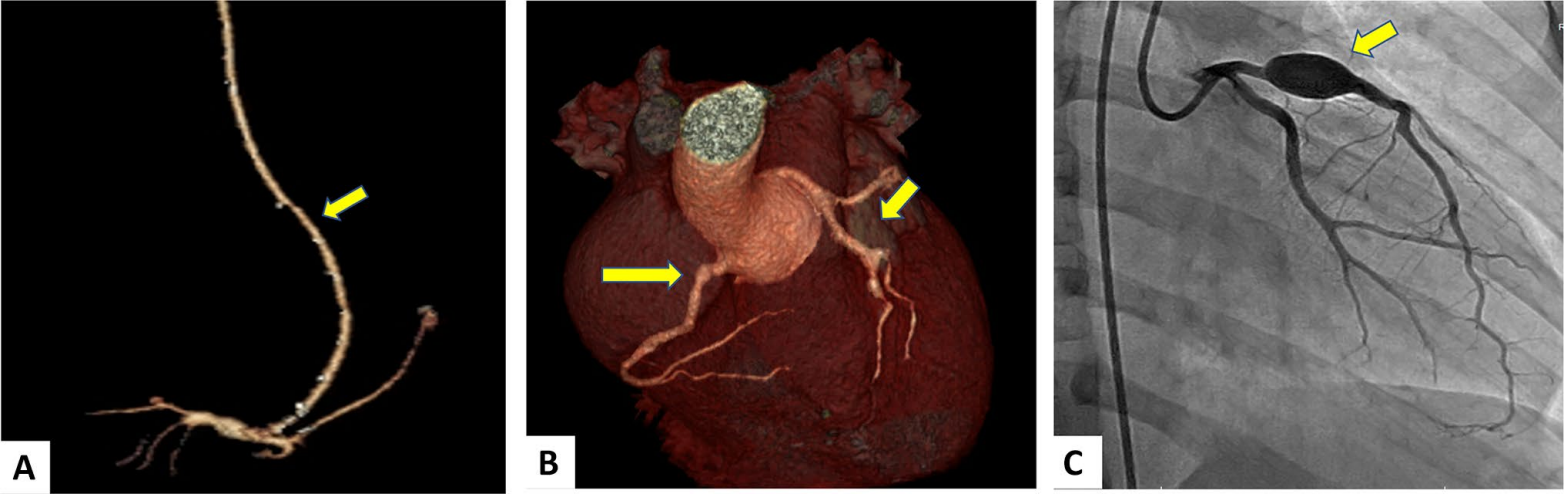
Coronary artery imaging in three patients with abnormal stress echocardiograms. A Patient 1 with CTA performed in 14 years after KD diagnosis demonstrating a patent RIMA to RCA bypass (yellow arrow). B Patient 2 with CTA performed 9 years after diagnosis demonstrating multiple coronary aneurysms (yellow arrows). C Patient 3 with cardiac catheterization performed 6 years after diagnosis demonstrating a giant coronary aneurysm in LAD (yellow arrow)
